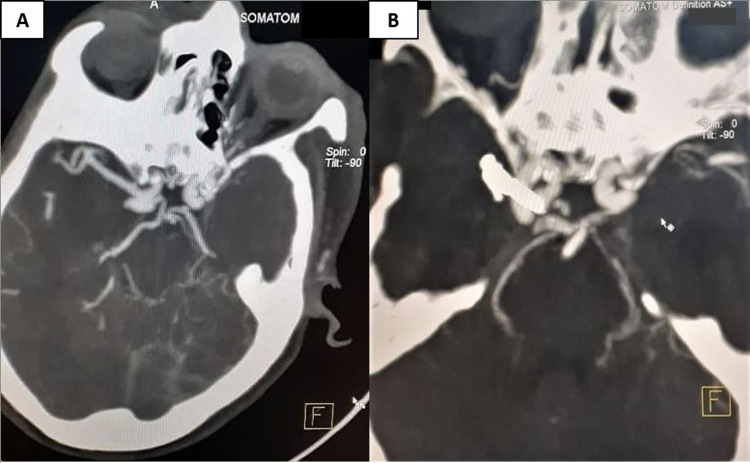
(A) Preoperative computed tomography angiography showing right paraclinoid ruptured aneurysm and (B) postoperative computed tomography angiography of the same patient showing clips in situ with complete occlusion of aneurysm
